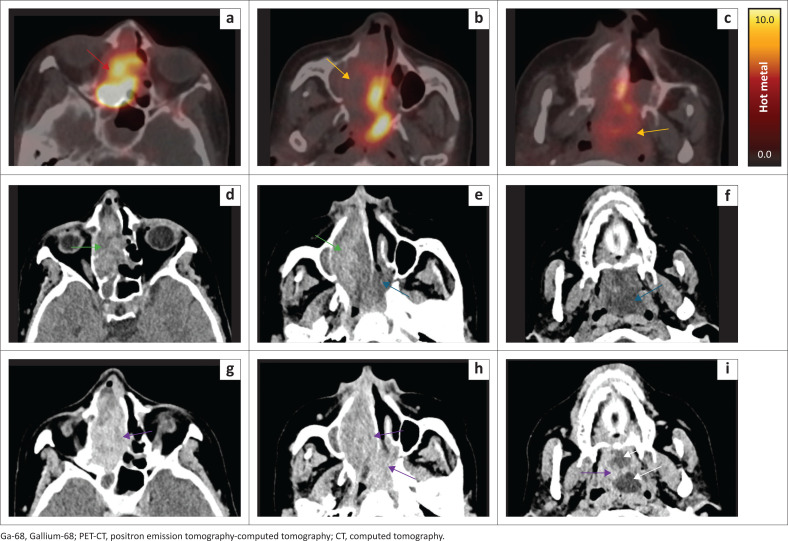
(a–c) Ga-68 DOTANOC PET-CT (d–f) Uncontrasted CT (g–i) Post-contrast CT. Multistudy axial imaging through the paranasal sinuses from superior to inferior, at corresponding levels, showing a soft tissue mass in the right ethmoid sinuses and nasal cavity extending posteriorly into the oro-nasopharynx. (a–c) Intensely DOTANOC avid mass (red arrow) with photopaenic lateral nasal passage and nasopharyngeal components (orange arrows). (d–f) Heterogenous appearance on uncontrasted CT with both hyper- (green arrows) and hypo- (blue arrows) attenuating components. (g–i) Post-contrast enhancement (purple arrows) with residual areas of hypoattenuation in the nasopharynx (white arrows).
